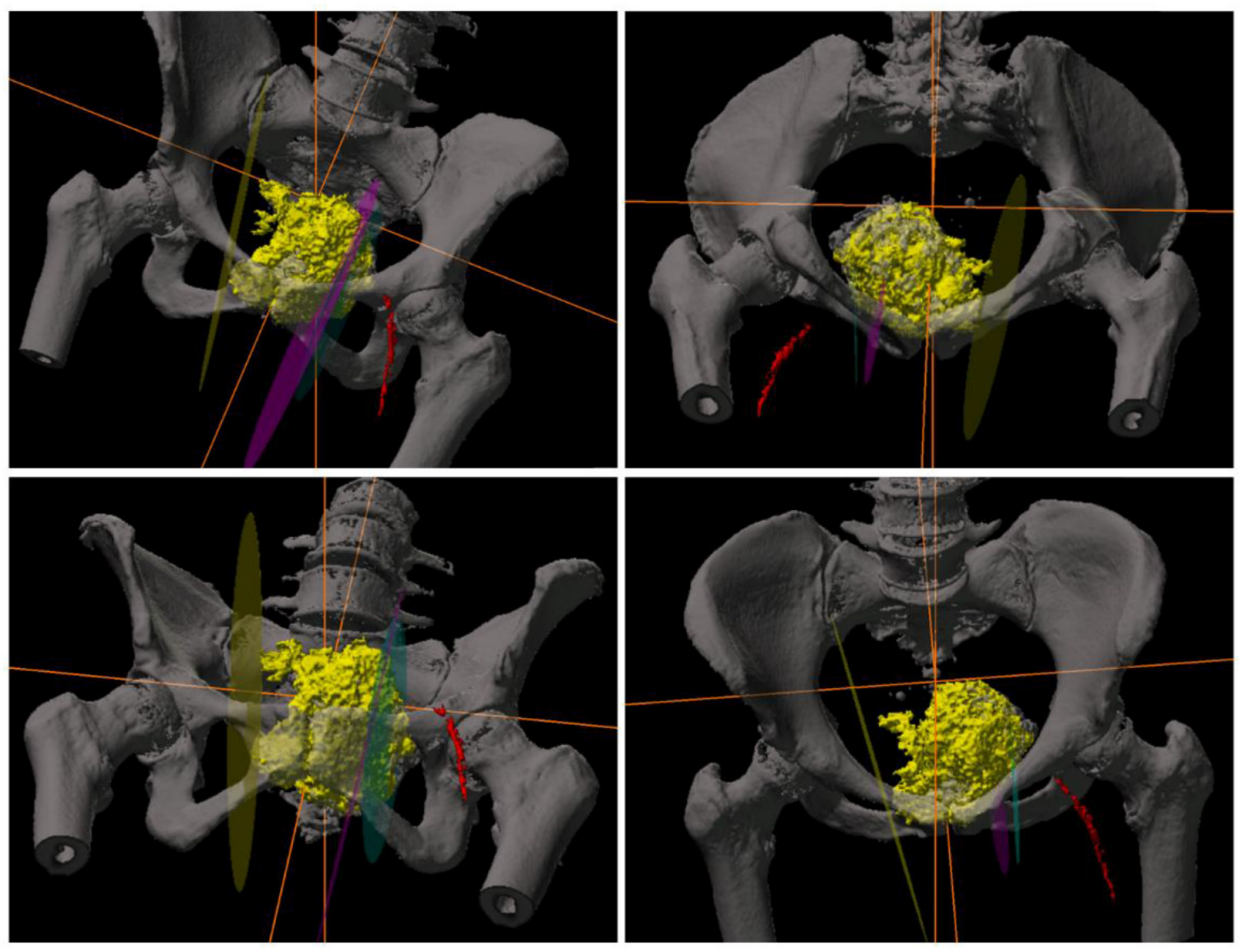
3D-CT reconstruction images of the pelvis demonstrating the tumor and computer navigation-system utilized intra-operatively.
